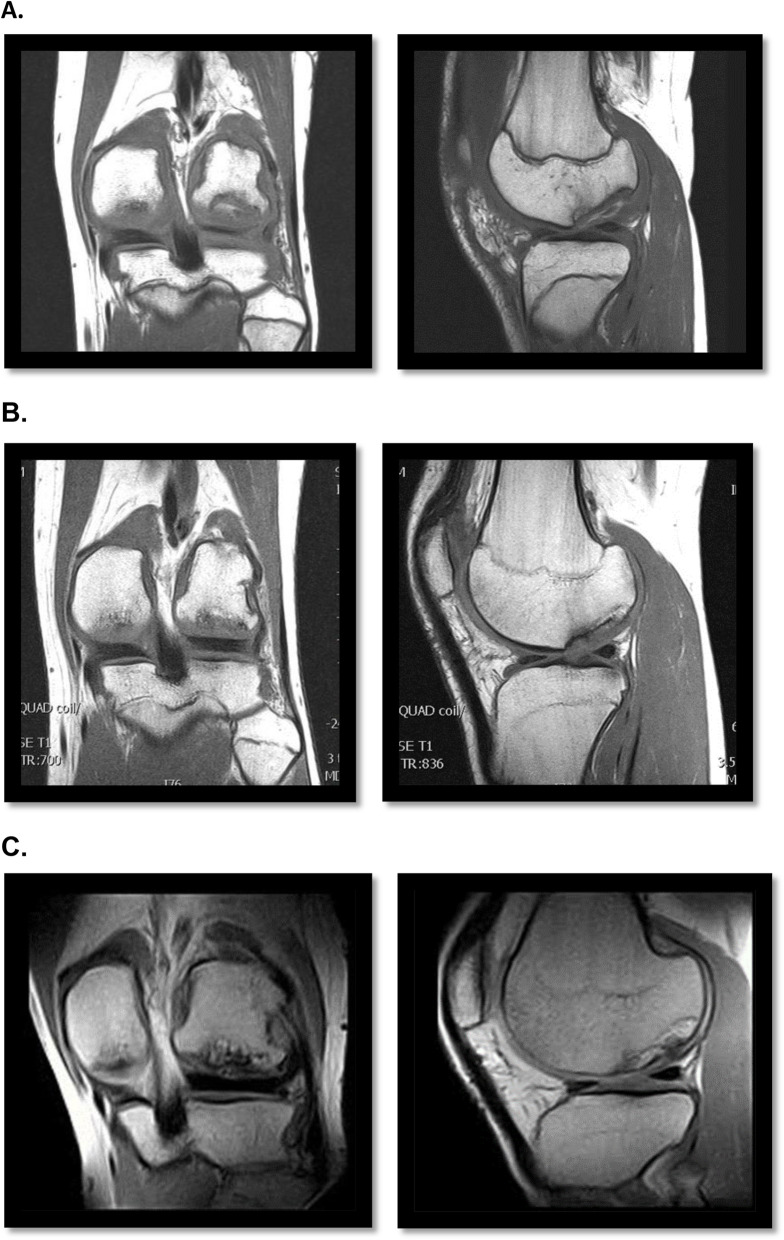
Sample case. Magnetic resonance imaging (coronal and sagittal view) of an osteochondritis dissecans on the lateral femoral condyle of the left knee in a 15 years old male treated with Maioregen® osteochondral scaffold. a Pre-operative; b 12 months follow-up; c 5 years follow-up
